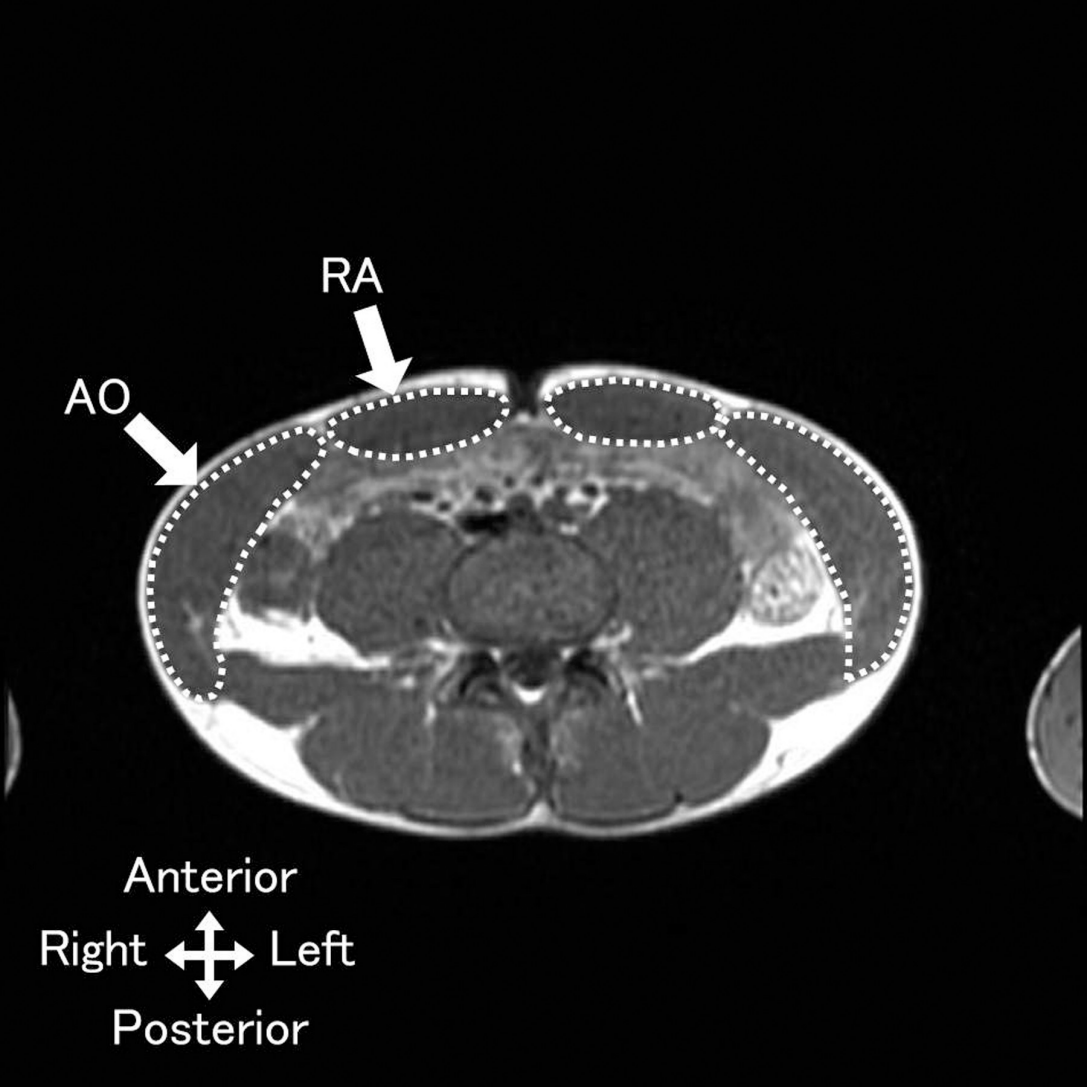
A typical magnetic resonance image of the trunk. The white broken lines are the borders of muscles. RA, rectus abdominis; AO, abdominal oblique muscles (internal and external obliques and transversus abdominis).
